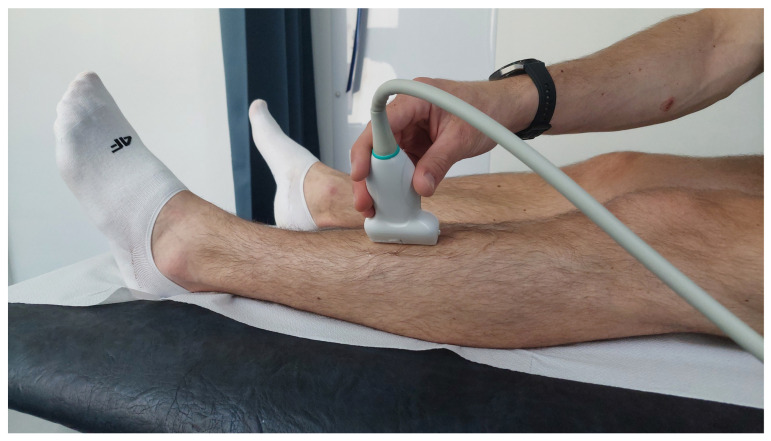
Standardized participant positioning and ultrasound transducer placement during SWE assessment of the tibialis anterior muscle.
